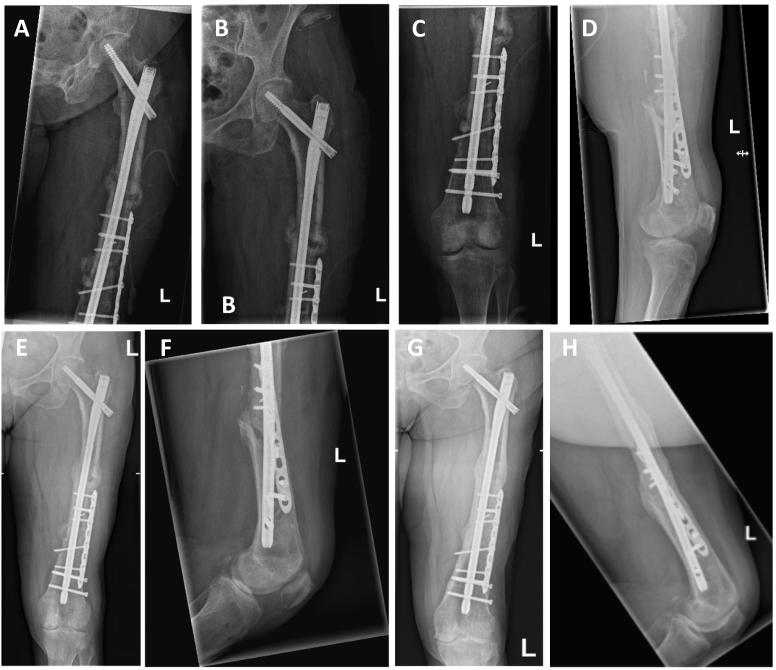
Postoperative ap (A + B) and lateral (C + D) X-rays after removal of the antibiotic beads, re-debridement and re-osteosynthesis with silver-coated gamma nail and augmentation plate. X-rays at 3 weeks after re-osteosynthesis (E + F). X-rays at 26 months after re-osteosynthesis with full consolidation of the proximal fracture and the distal non-union site without implant failure (G + H).
